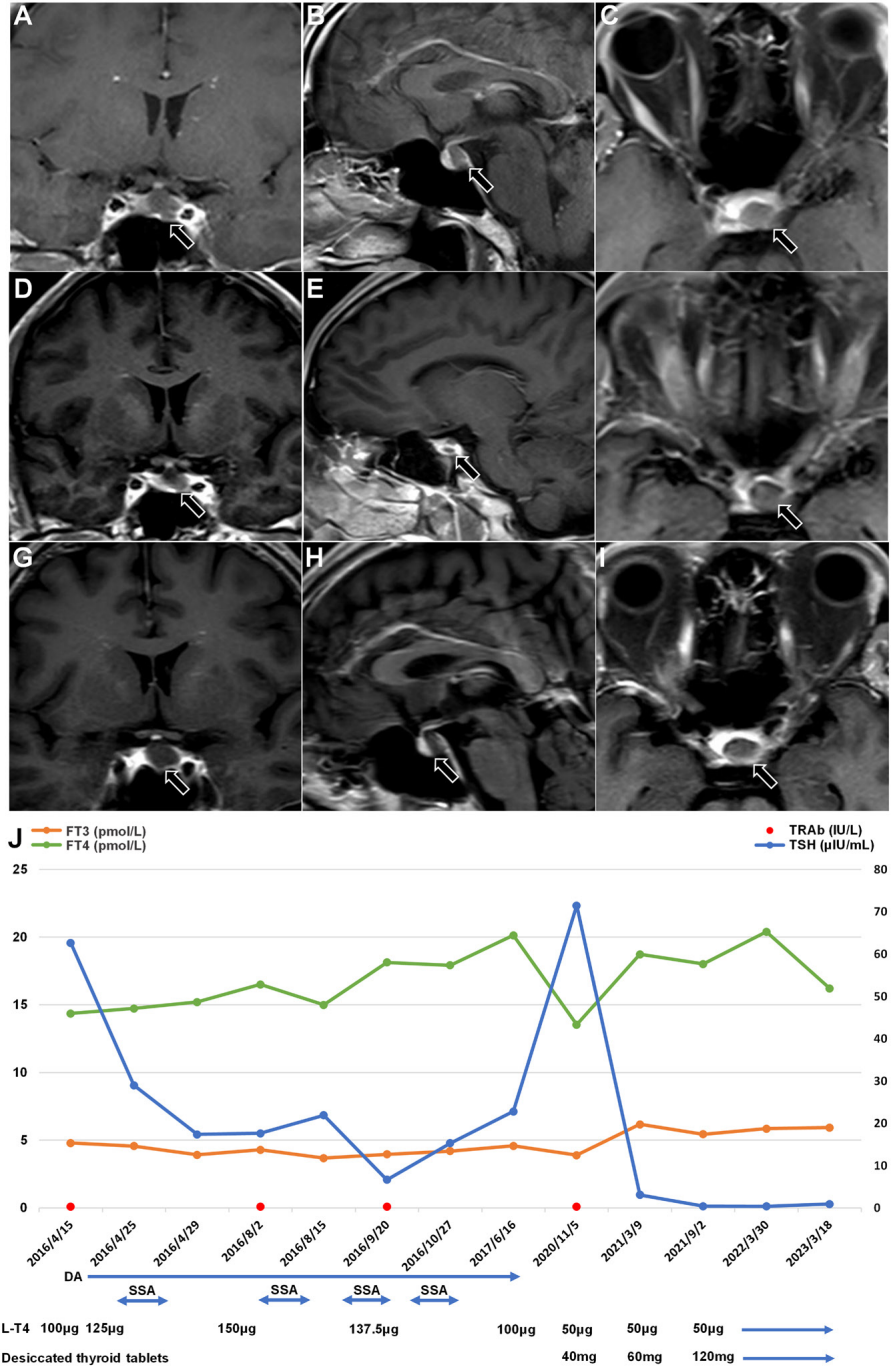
Pituitary-enhanced MRI, thyroid function change, and treatment of case 4. DA, dopamine receptor agonist; FT3, free tri-iodothyronine; FT4, free thyroxine; L-T4, levothyroxine; SSA, somatostatin analog; TRAb, thyroid-stimulating hormone receptor antibodies; TSH, thyroid-stimulating hormone; TSS, transsphenoidal surgery. Normal ranges: FT4 7.64–16.03 pmol/L, FT3 3.28–6.47 pmol/L, TSH 0.49–4.91 μIU/mL, TRAb 0–1.7 IU/L.
